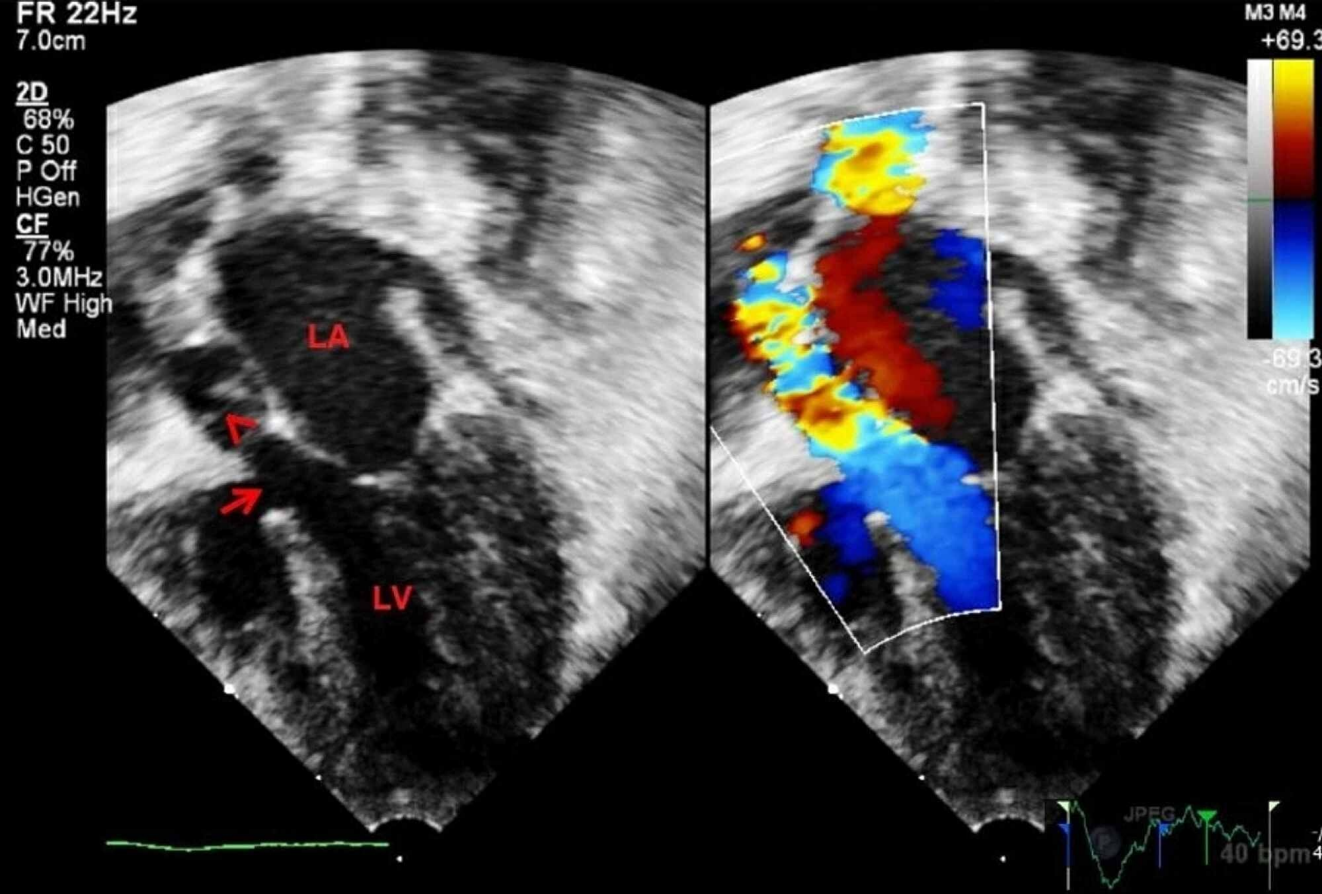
Two dimensional and color flow mapping of apical five chamber view showing outlet subaortic ventricular septal defect (arrow), smallish left ventricular outflow, and turbulent flow across stenotic aortic valve (arrow head). LA, left atrium; LV, left ventricle
